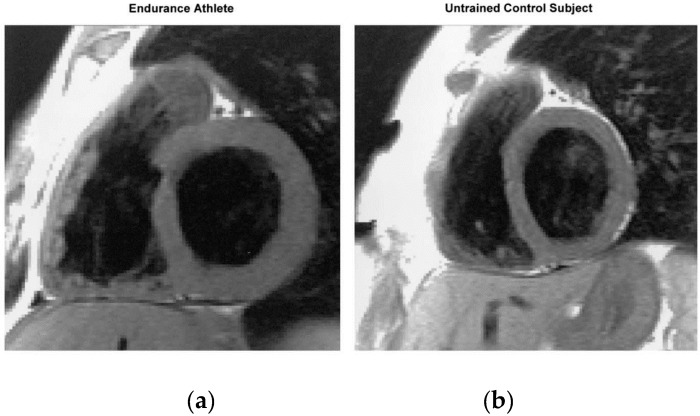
End-diastolic T1-weighted short-axis slice from an endurance athlete (a) and an untrained control subject (b). Compared with the heart of the control subject, the endurance athlete’s heart is characterized by an enlarged volume and a greater myocardial mass of both ventricles, while the proportions of the left and right heart are the same as in the untrained control subject. Taken with permission from Scharhag et al. [26].
